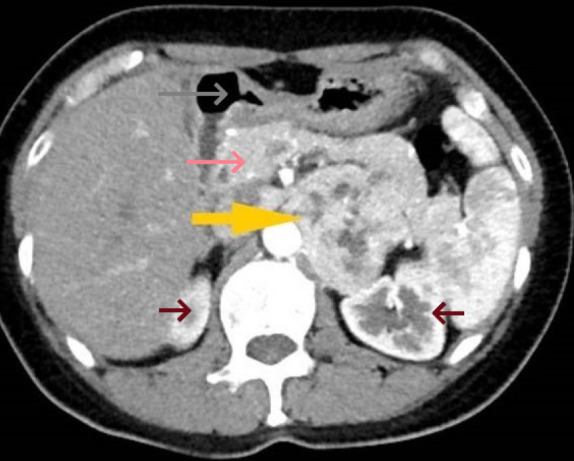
Two-dimensional CT Image of Pheochromocytoma (the tumor is indicated by a yellow arrow, the gastrointestinal tract is indicated by a brown arrow, the kidney is indicated by a purple arrow, and the pancreas is indicated by a pink arrow).
